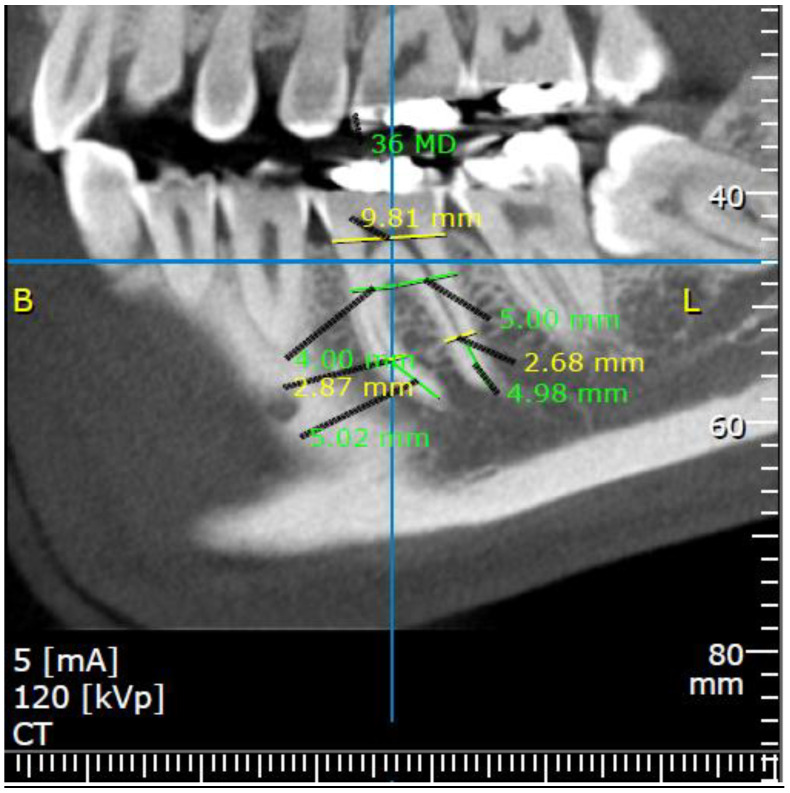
Mesiodistal (MD) aspect measurements of left first molar, assessed in 3 different points, referred to tooth long axis: (1) A—5 mm coronal to the apices (mesial root: 5.02 mm from the apex; green line, the MD width is 2.87 mm; yellow line); (distal root: 4.98 mm from the apex; green line, the MD width is 2.68 mm; yellow line), (2) F—at furcation level (MD width is 4 mm for mesial root and 5 mm for distal root), (3) X—alveolar crest level (MD width is 9.81mm). CBCT para-axial sagittal section.
